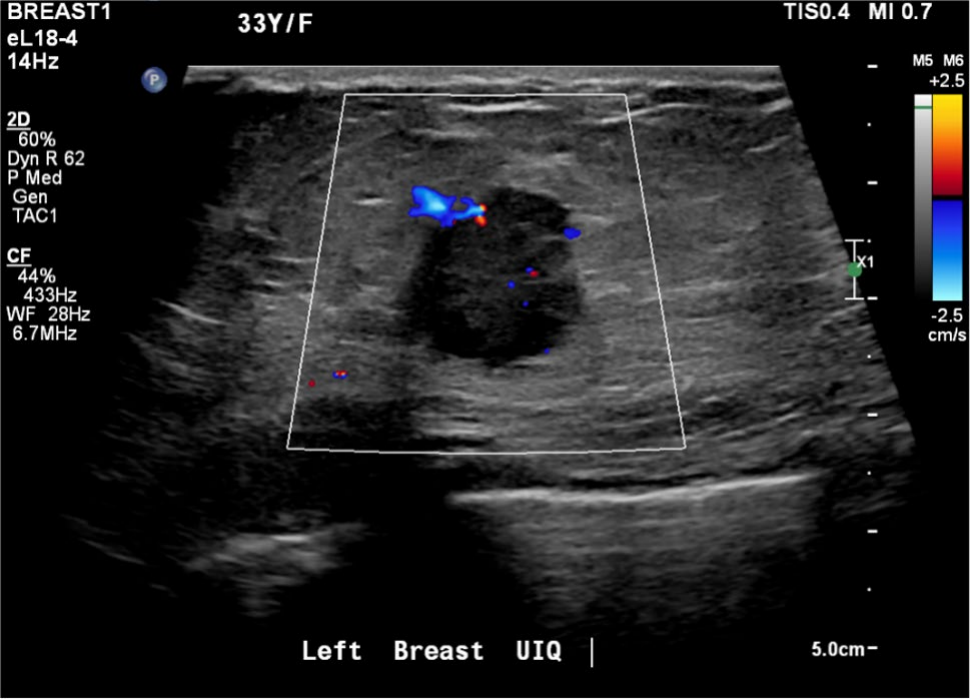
An ultrasound for a 33-year old female shows a round mass with angular margins
